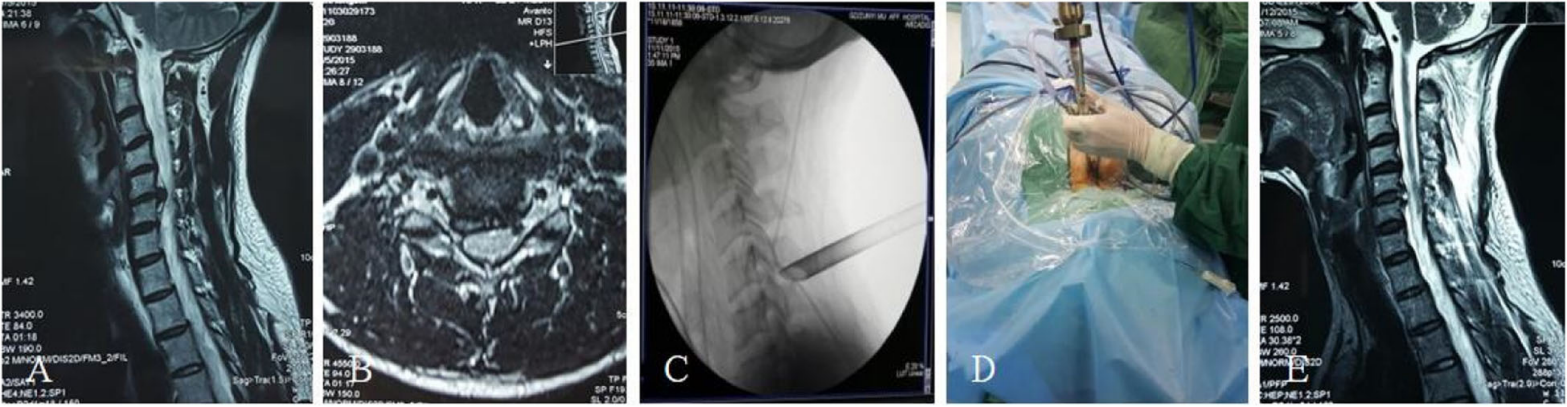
Percutaneous posterior full-endoscopic discectomy and nerve root decompression. a The MRI sagittal image of the cervical spine showed C5/6 cervical disc herniation with local spinal stenosis. b The MRI axial image of the cervical spine showed C5/6 posterolateral cervical disc herniation with spinal nerve compression. c Intraoperative radiography. d Intraoperative view. e Postoperative review of cervical spine MRI sagittal image showed complete removal of the C5/6 herniated disc tissue and complete decompression of spinal nerve
